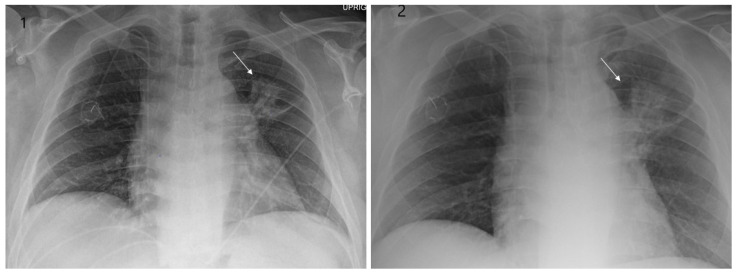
Chest X-ray showing radiation pneumonitis Image 1—Frontal chest X-ray showing left upper lobe mass (arrow), the patient also had a right internal jugular port placed. Image 2—Post radiation treatment frontal chest X-ray showing increasing alveolar and interstitial opacities in the left upper lobe and in the left lower lobe in a patient suspected of radiation pneumonitis.
